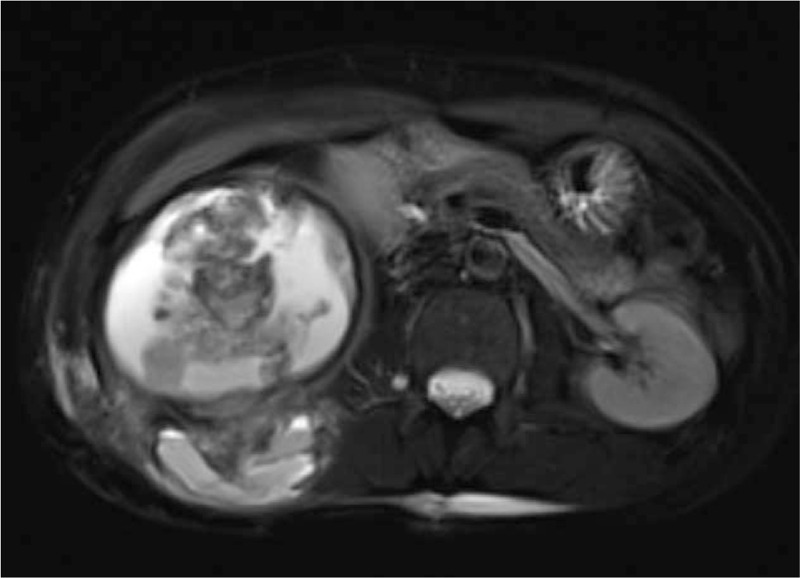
MRI: T2WI mass showed high and low mixed signals, but mainly high signals in the cystic area.
